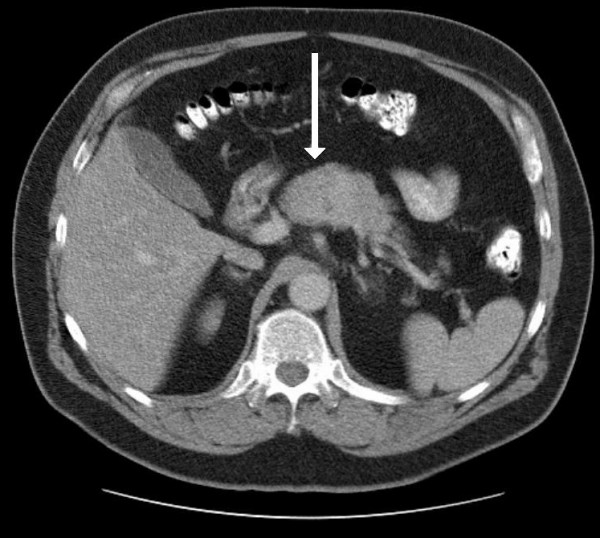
CT scan of the abdomen with contrast. A heterogeneously enhancing, solid mass can be identified at the junction of the head and body of the pancreas (arrow).
